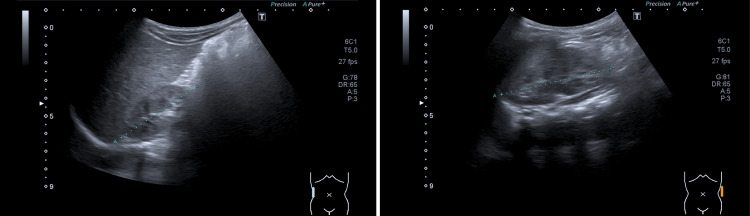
Bilateral renal ultrasound performed at 12 months of age. Normal-sized kidneys with preserved corticomedullary differentiation.
